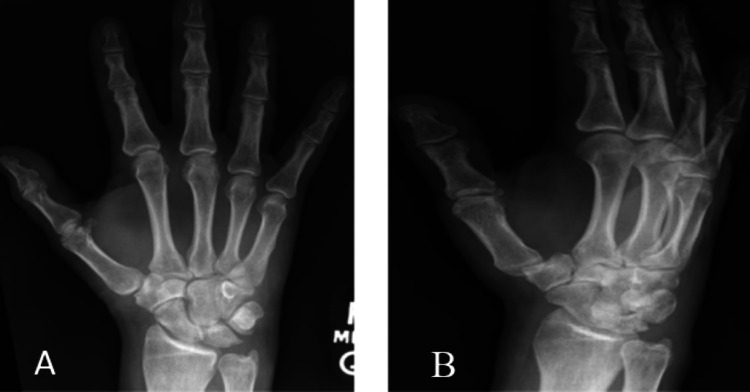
(A) Frontal and (B) lateral view. X-ray images of the hand at the initial presentation.
